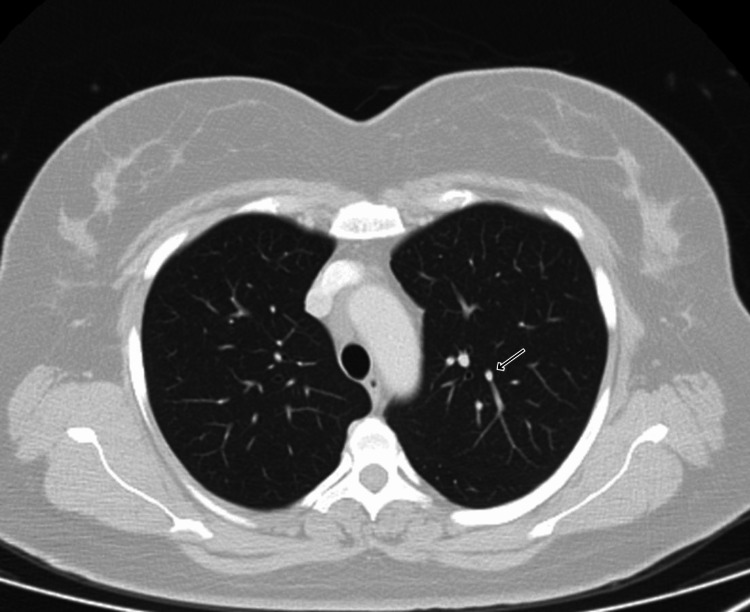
CT chest showing multiple pulmonary nodules.
